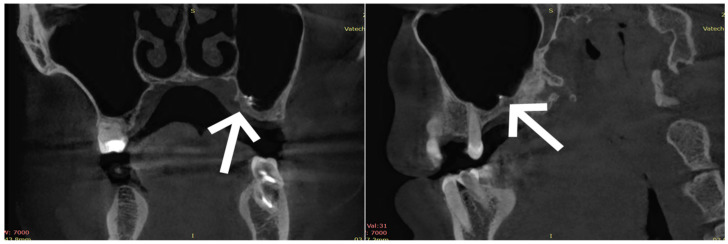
A CBCT scan revealed a radiopaque foreign body in the left maxillary sinus (arrow).
